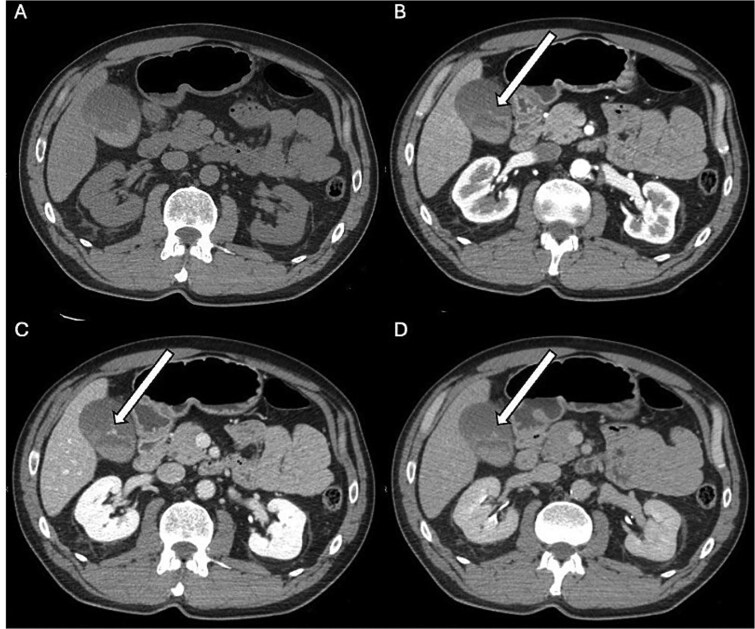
Initial multiphasic CT scan. (A) Non-contrast axial image showing the gallbladder containing spontaneously hyperdense material, consistent with blood. (B) Arterial phase, (C) venous phase, and (D) delayed phase. In these contrast-enhanced phases, focal contrast extravasation ("blush") is observed pooling within the lumen of the gallbladder body (arrow); this finding, best appreciated in the venous phase (C), is consistent with active bleeding.
